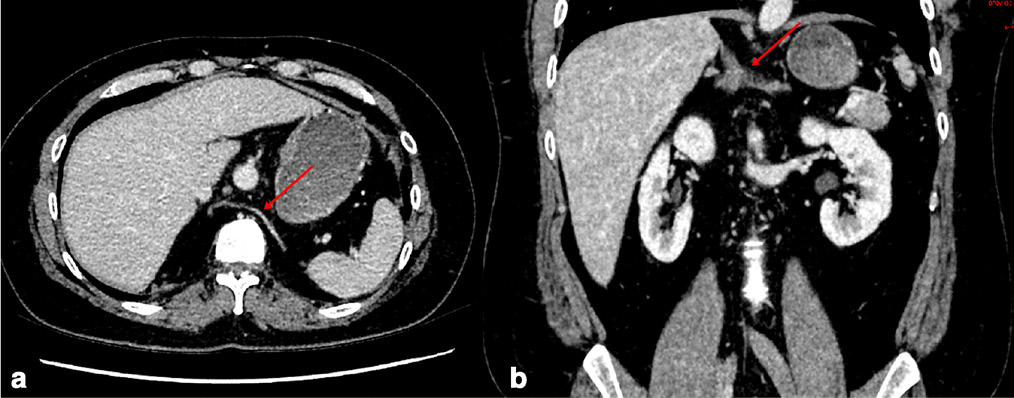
(a) Axial and (b) Coronal reformats of a CT abdomen and pelvis scan with contrast in the portal venous phase demonstrating the unique horseshoe-shaped fused adrenal glands (red arrows).
